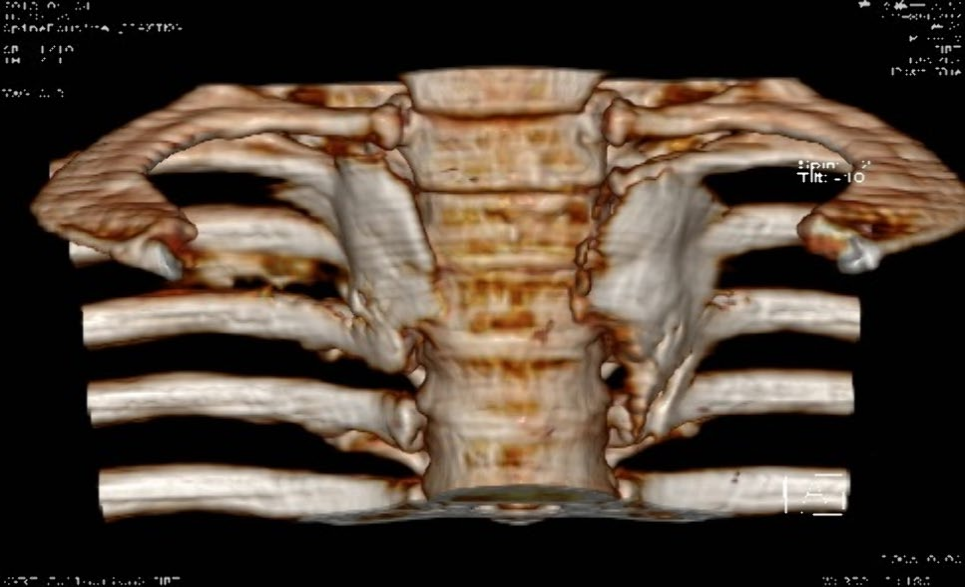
Thoracic sympathetic anhydrous alcohol block(T4)—Three-dimensional reconstructionChemical lumbar sympathetic nerve blockThe lumbar sympathetic nerve block can also be performed under local anesthesia [50]. The tip of the puncture needle was guided by CT to the anterolateral part of the L3 vertebral body and the anterior part of the psoas major muscle, and 3 mL of 1% lidocaine containing the contrast agent iodohyl was injected into the lumbar sympathetic nerve. It was observed on the CT scan that the injected liquid in the lumbar segment was distributed between the psoas major muscles on both sides and the L2 and/or L3 vertebral body (Fig. 3). When the PI of the patient's toes increased by more than five times and the temperature of the soles of the feet increased by more than 3℃, 5 m of anhydrous ethanol containing the contrast agent iodihexyl alcohol was injected into the lumbar puncture site. CT scan was repeated, and a three-dimensional reconstruction was performed to observe the distribution of the drug solution (Fig. 4). The operation was completed when the drug solution covered the anterior sides of the L2 and L3 vertebrae. During lumbar sympathetic neurochemical destruction (L2 is more common), anhydrous ethanol diffuses up to the lumbar 1 level, leading to ejaculation disorders. In addition, the diffusion of anhydrous ethanol can damage the sciatic nerve and even lead to permanent damage to the lateral femoral cutaneous nerve [51].
